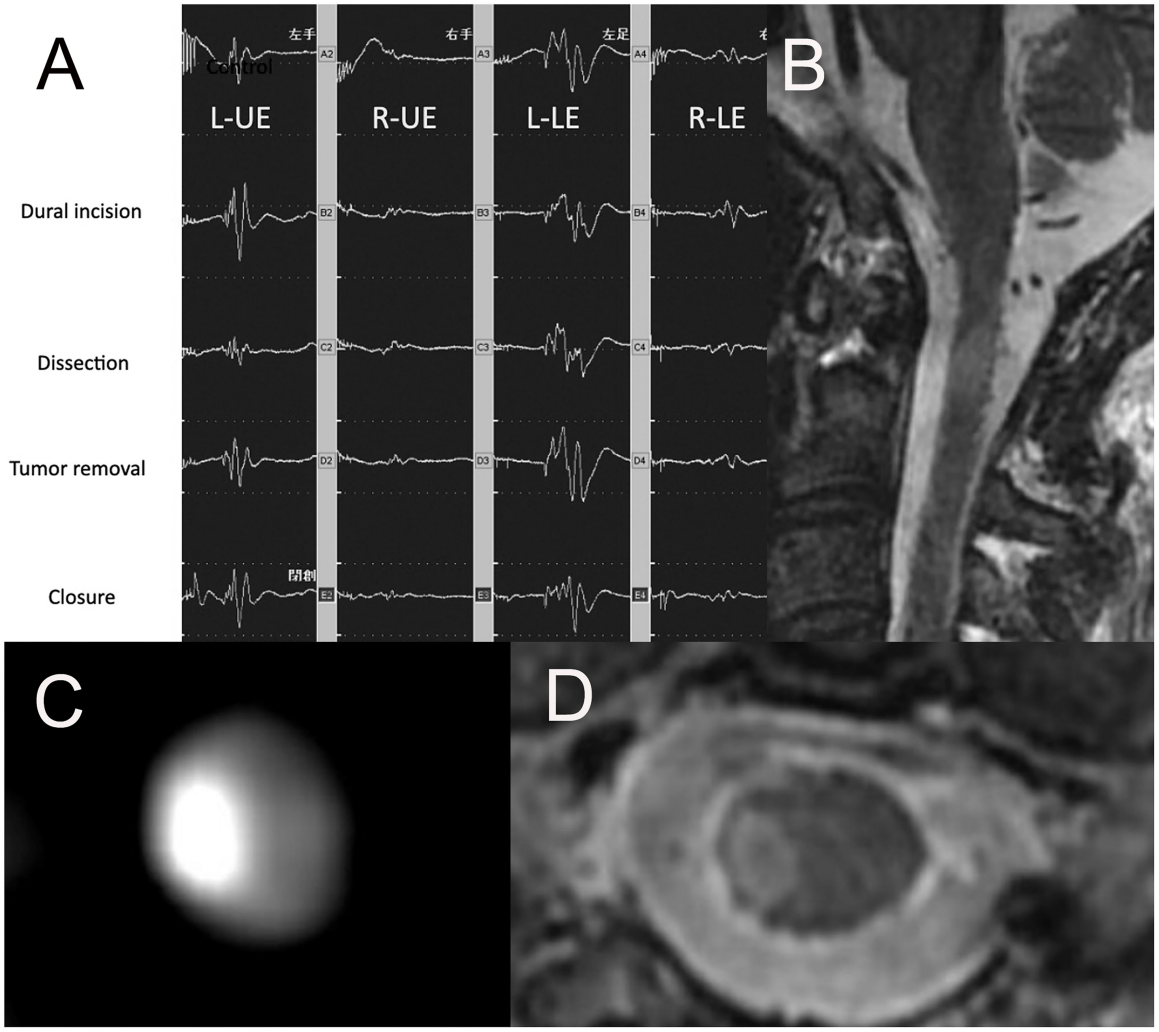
(A) Ratio motor-evoked potential (MEP) of right UE and right LE suddenly reached down to 0 and 30% compared to the initial value, (B) the sagittal image of postoperative T1WI of MRI, (C) the axial image of postoperative DWI of MRI, (D) the axial image of postoperative FLAIR of MRI. UE, upper extremity; LE, lower extremity; TIWI, T1-weighted image; MRI, magnetic resonance imaging; T2WI, T2-weighted image; DWI, diffusion-weighted imaging; FLAIR, fluid-attenuated inversion recovery.
